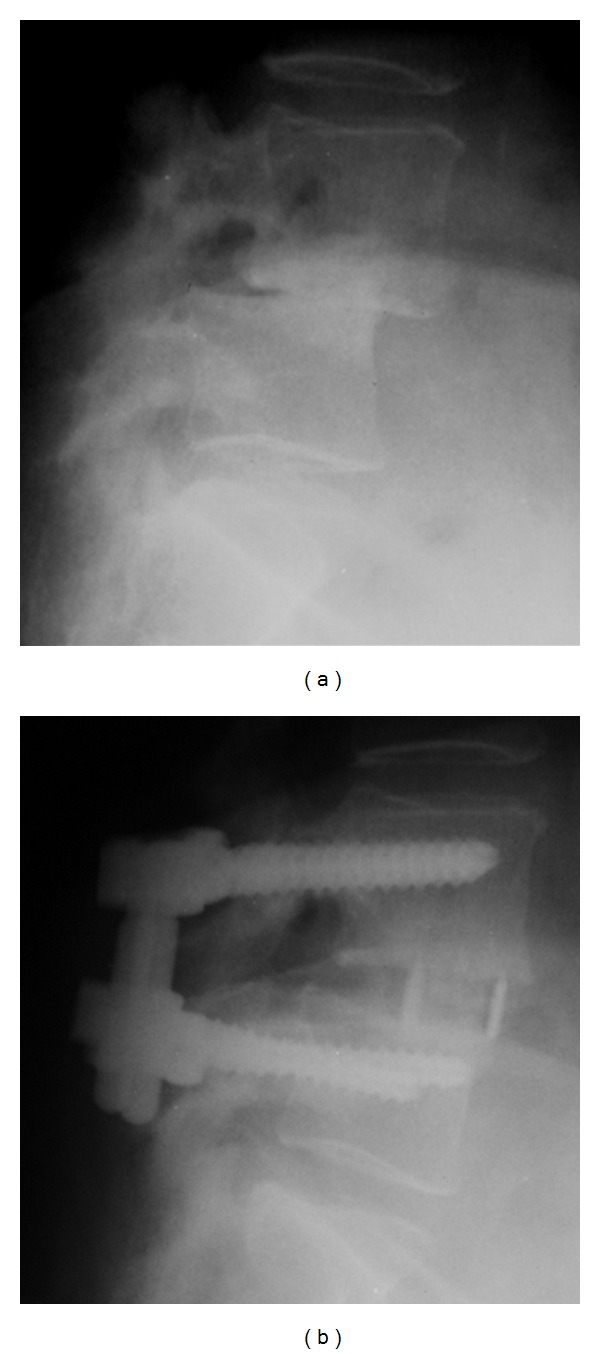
(a) Preoperative lateral radiograph. (b) Lateral radiograph 12-months postoperative.
